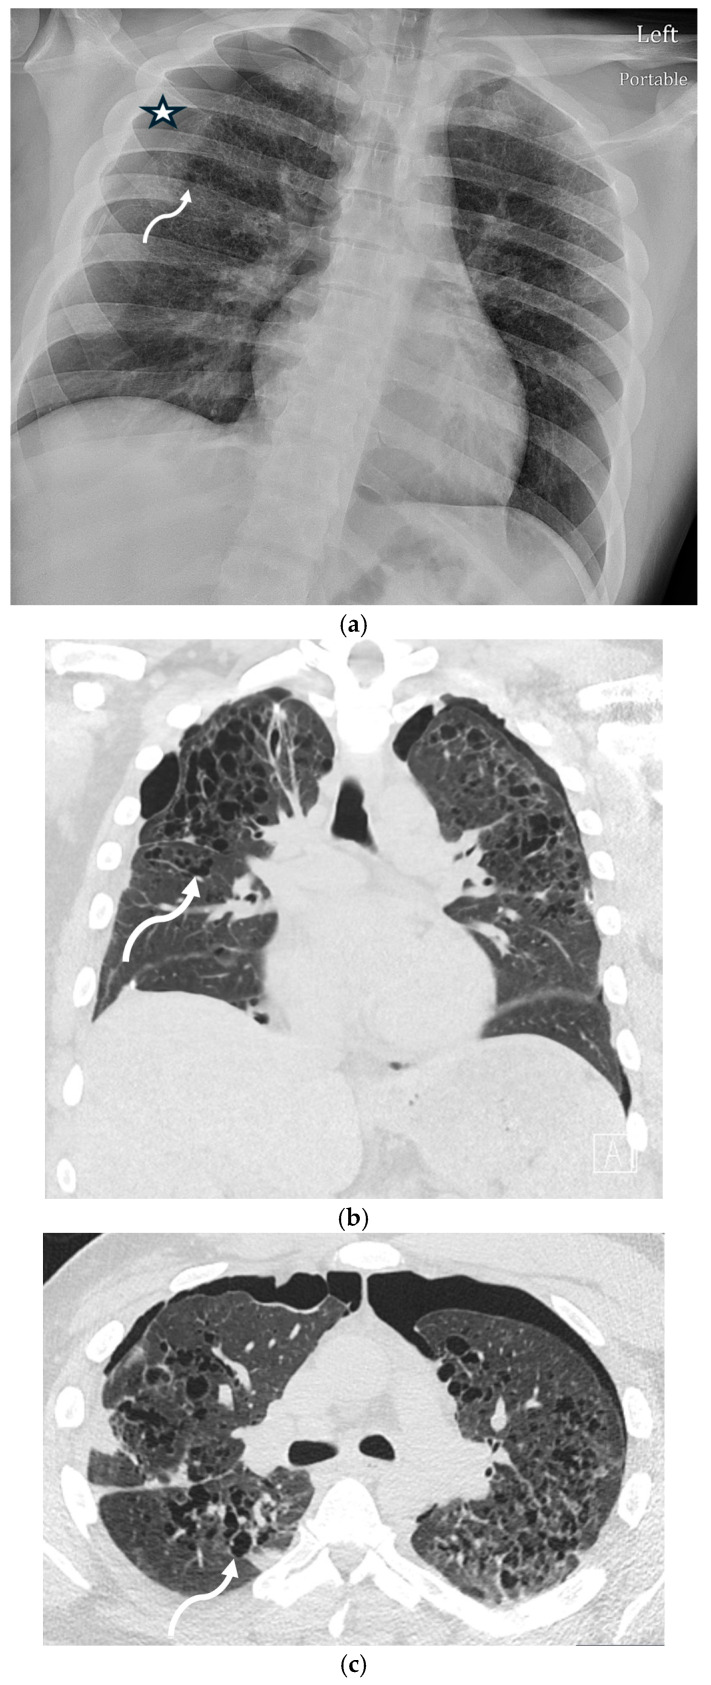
Pulmonary Langerhans cell histiocytosis in a 26-year-old male. (a) Frontal radiograph shows a small right pneumothorax (star) and upper-lobe interstitial and cystic changes (curved arrow). (b,c) Coronal and axial CT images confirm upper-lobe predominant, bizarre-shaped cysts (curved arrows) with associated interstitial thickening, moderate left pneumothorax, and small loculated right hydropneumothorax.
